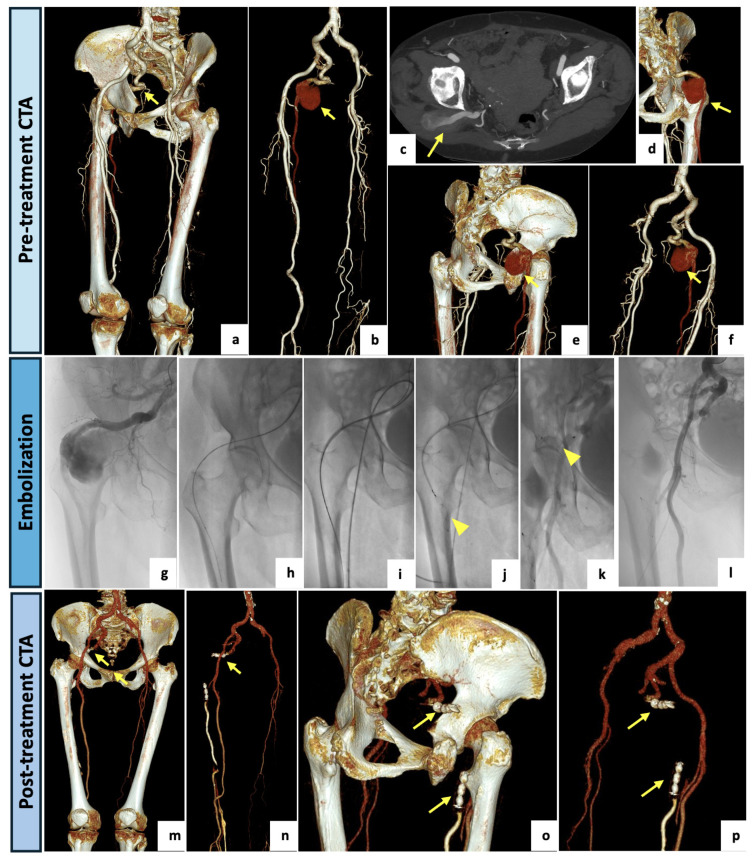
(a) VR reconstruction of the preoperative CTA showing a right PSA originating from the internal iliac artery (arrow), continuing as a popliteal artery and associated with a normal SFA (type 1). (b–f) Three-dimensional reconstructions, with and without bone structures, and axial maximal intensity projections showing a huge aneurysmal degeneration (57 mm in the AP caliber) of the PSA in the posterior aspect of the thigh (arrows). (g) Intraprocedural selective angiography of the right PSA performed after obtaining a left humeral access with a 4F-sheat (60 cm), confirming a large aneurysm. (h,i) Intraprocedural images obtained after right common femoral artery access with a 6F-sheat and right PSA selective catheterization. (j,k) Aneurysm exclusion with “sandwich technique”: embolization through two vascular plugs deployed in the outflow and in the inflow vessel (arrowheads). (l) At final angiography, a nearly complete occlusion of the aneurysm (small residual slow flow) was documented. (m–p) VR reconstruction of postoperative CTA (1-month), demonstrating the complete exclusion of the PSA aneurysm. Both inflow and outflow were occluded by vascular plugs (arrows).
